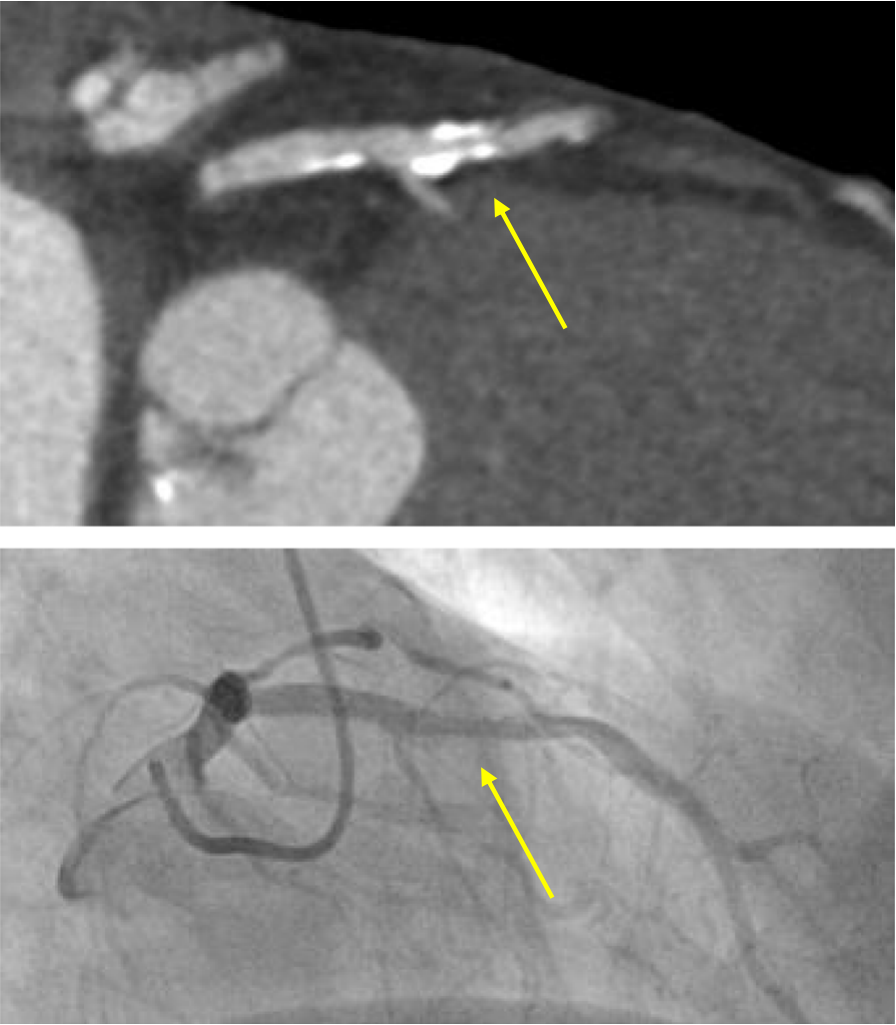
In this example, the mid-left descending coronary artery lesion (yellow arrow) is calcified. Blooming artifact in the CT image results in overestimation of lumen stenosis in this area. Invasive coronary angiography (ICA) revealed a less severe stenosis than perceived on CT. Fractional fluid reserve for this lesion was 0.82. Image credit: Centro Cardiologico Monzino (used with permission)
